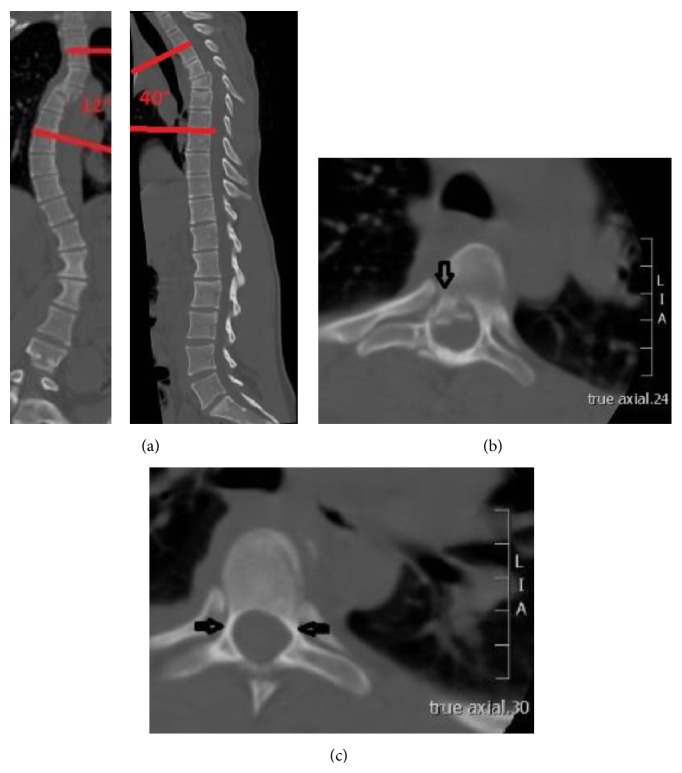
(a) CT scans showing the preexisting scoliosis and the fracture of T5. Cobb angle (T3–7): 12°, kyphosis angle (T3–7): 40°. (b) CT scan showing the fragment narrowing the spinal canal. (c) CT scan showing the small pedicles (blind on the right side, 2.5 mm on the left).
